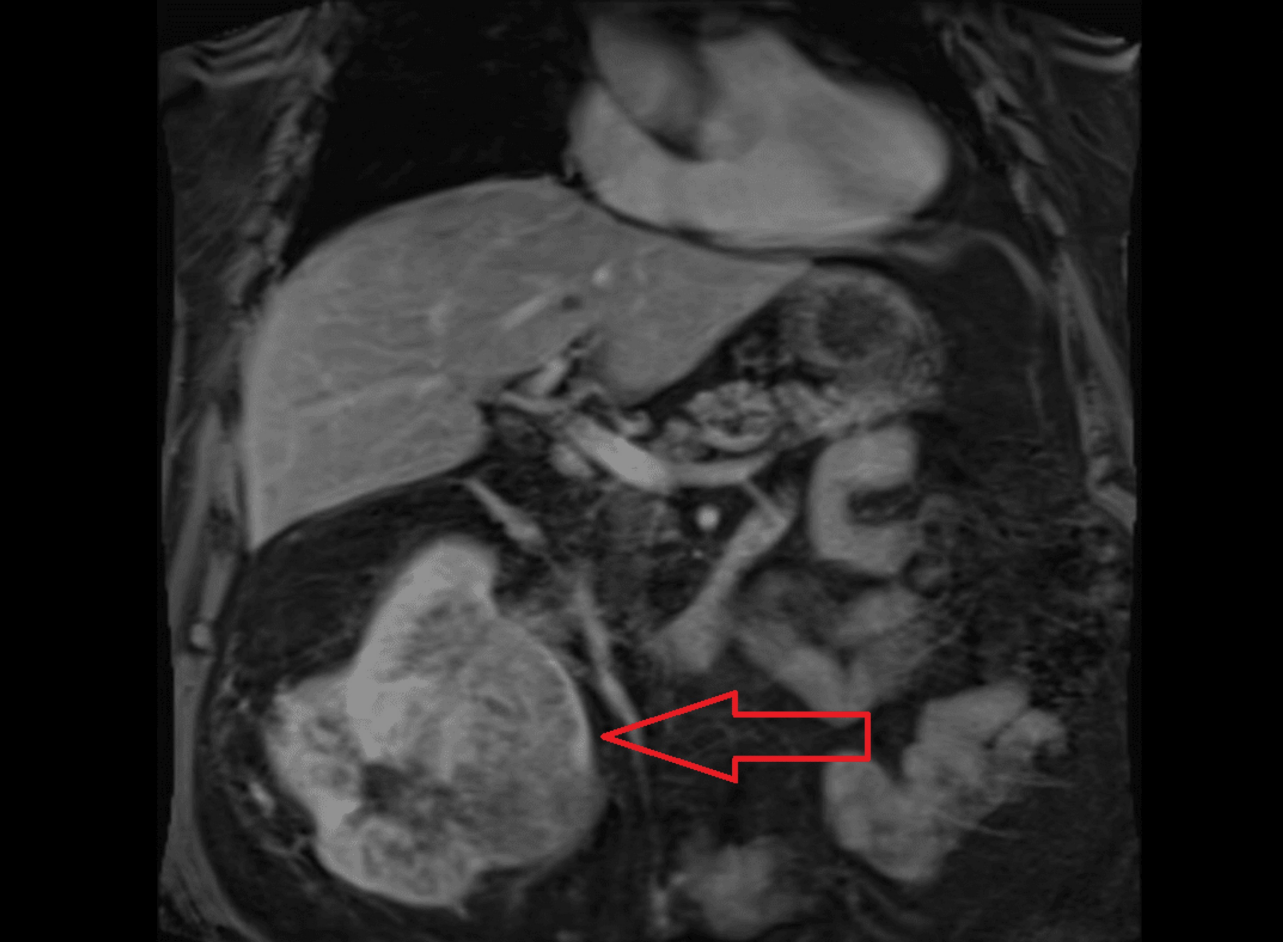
MRI Image Showing Renal Cell Carcinoma
